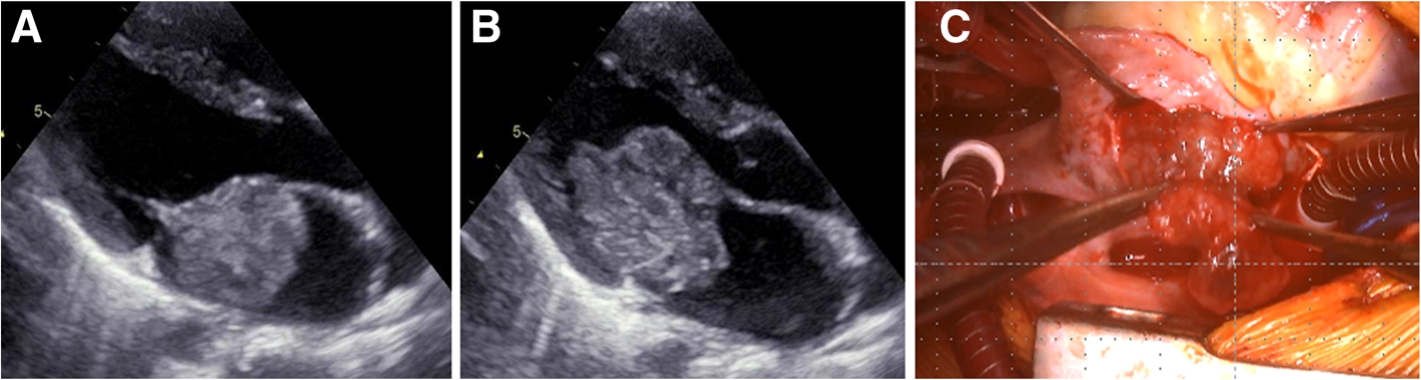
Echocardiography shows a giant mass in the left atrium (a), which prolapsed into the left ventricle during diastole (b). c A giant gelatinous tumor in the left atrium
